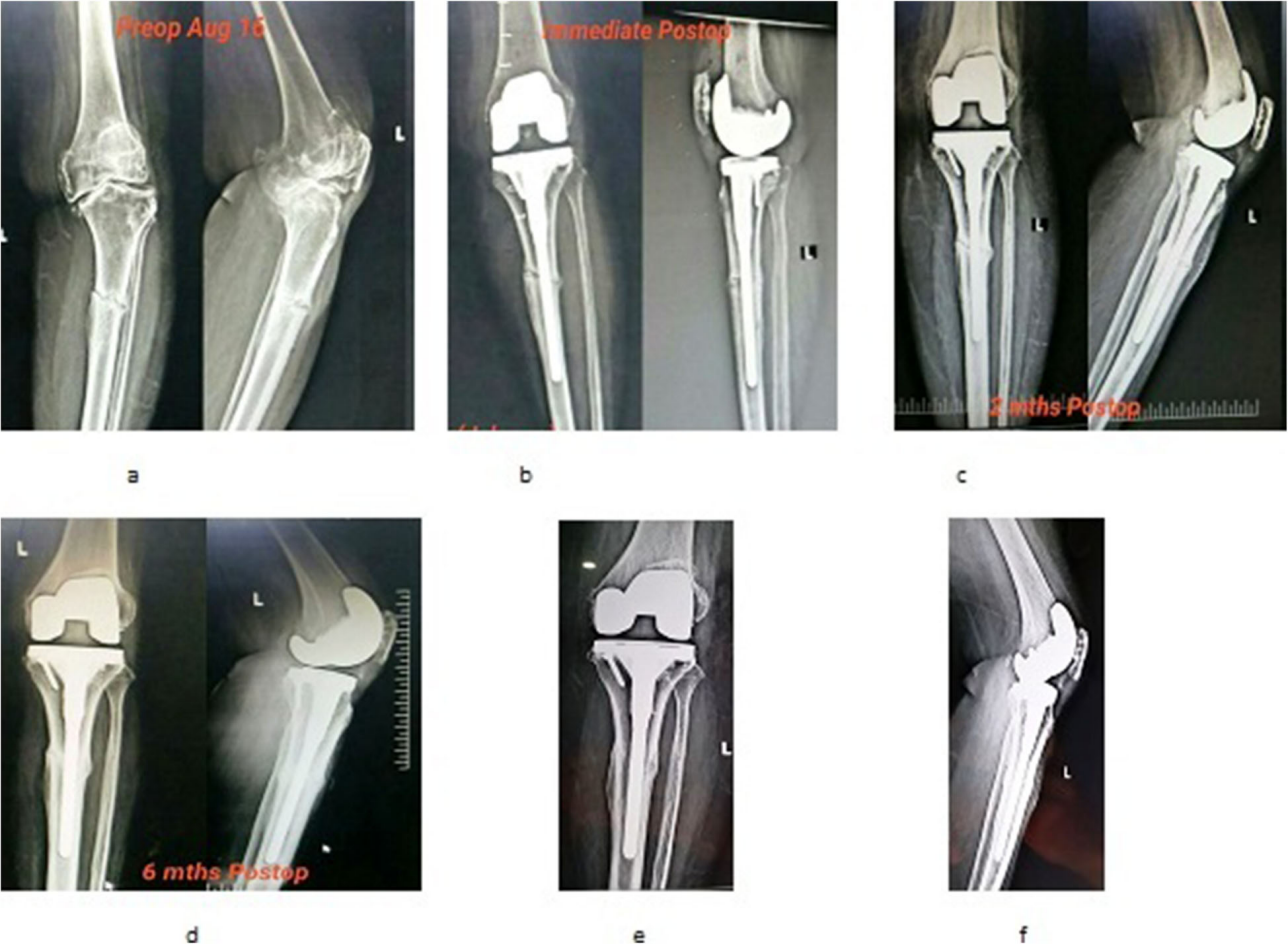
Left Knee X-rays (Delayed fracture healing) a) Preoperative anteroposterior and lateral view showing features suggestive of osteoarthritis, with deformity with stress fracture proximal tibia; b) Post operative X-ray anteroposterior and lateral view showing correction of deformity with modular stemmed knee prosthesis with intact fibula; c) 8 week follow-up anteroposterior and lateral view showing healing of fracture in process with correction of deformity with modular stemmed knee prosthesis with intact fibula; d) 24 week follow-up anteroposterior and lateral view showing healed stress fracture with correction of deformity with modular stemmed knee prosthesis with intact fibula; e) and f) Recent follow-up anteroposterior and lateral view showing healed stress fracture with correction of deformity with modular stemmed knee prosthesis in situ with intact fibula
